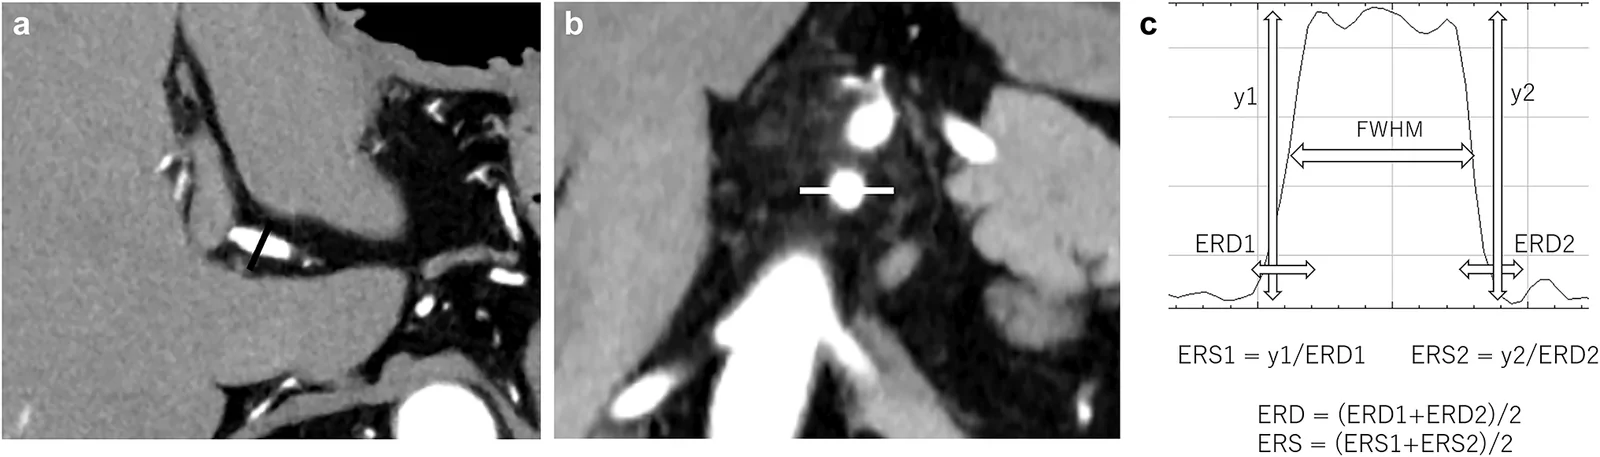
A linear region of interest was drawn perpendicularly across the PHA on the axial view (a) and SMA on the coronal view (b). Maximum computed tomography (CT) attenuation, full width at half maximum (FWHM), and slope at half maximum (average of the absolute values for the left and right slopes) were evaluated from the CT attenuation profile along the line regions of interest (c)
